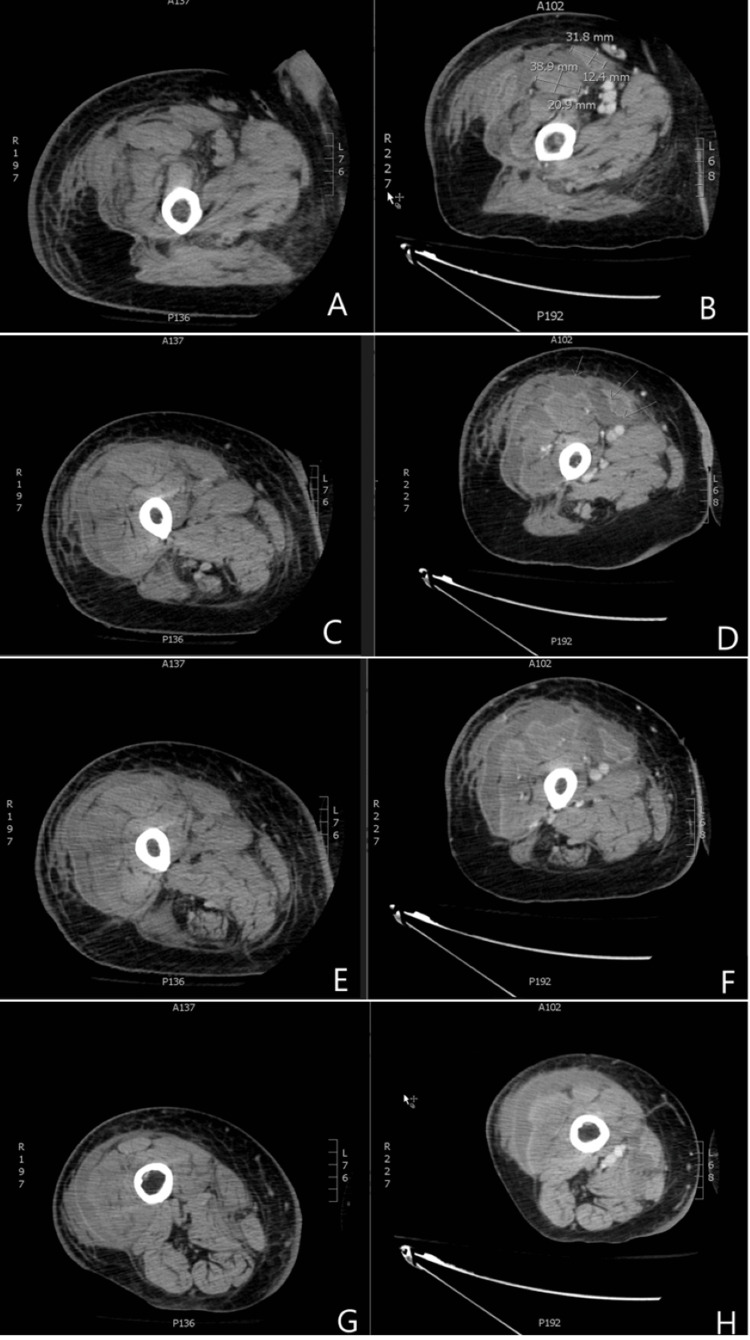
CT of the right femur #1 and #2 CT femur #1 (A, C, E, and G) without IV contrast and CT femur #2 (B, D, F, and H) with IV contrast were performed five days after CT femur #1. Transverse CT images of the right femur show cross sections descending from superior to inferior. The images on the left demonstrate initial findings that were suspicious of a soft tissue skin infection, and the images on the right were taken five days later, demonstrating the development of extensive necrotizing fasciitis. Images B and D show intra- and intermuscular abscesses with a concomitant reduction in muscle volume and size, namely the sartorius, rectus femoris, vastus medialis, vastus intermedius, and vastus lateralis. Images F and H show increasing rim-enhancing lesions of the medial compartment with necrosis of the adductor brevis, adductor longus, and adductor magnus. CT: computed tomography; IV: intravenous
